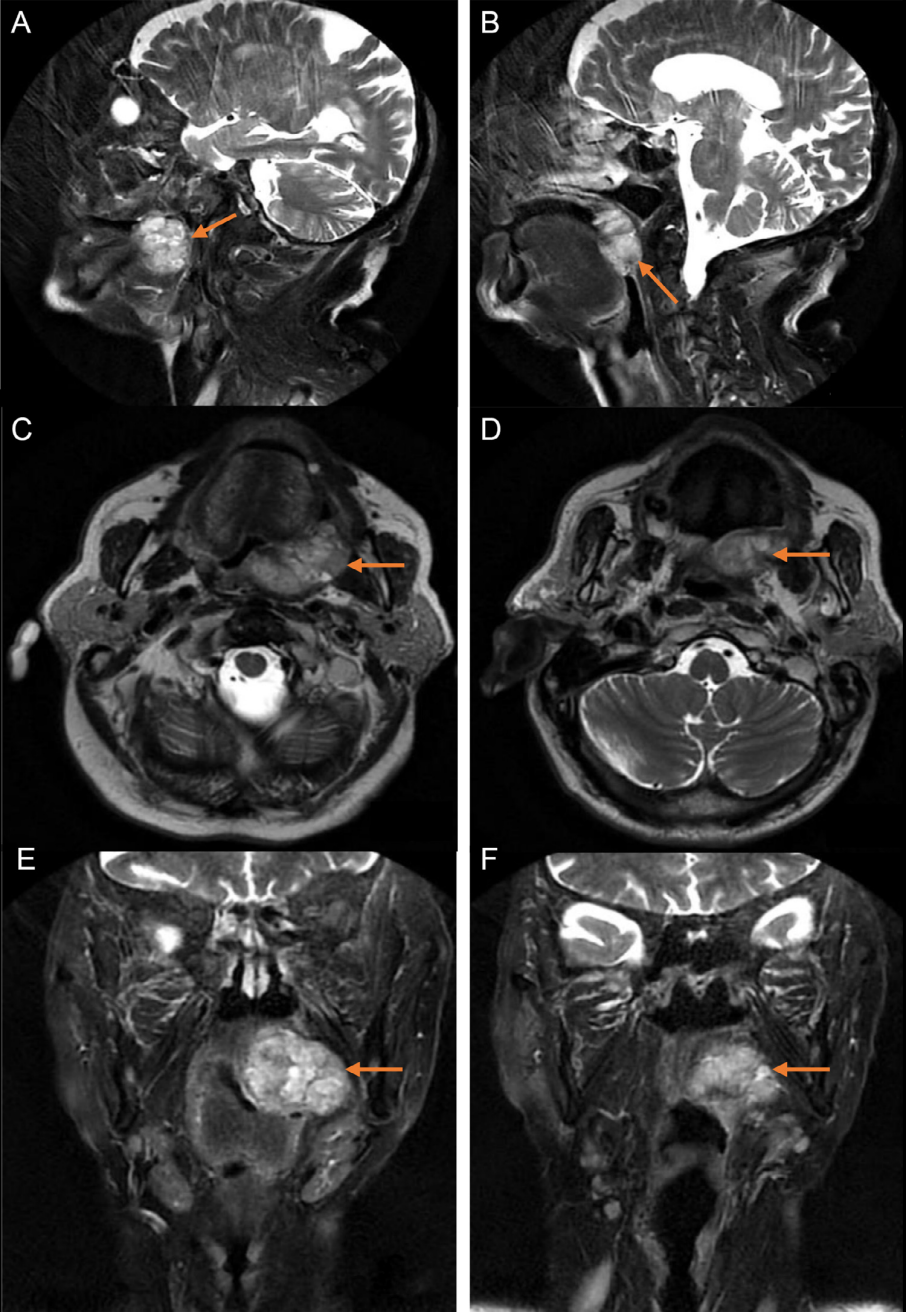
Initial head and neck MRI of our patient revealing a tumor of 39.4 × 21.4 × 39.7 mm (orange arrows). (A and B) Sagittal T2 propeller sequence. (C and D) Axial T2 propeller sequence. (E and F) Coronal T2 propeller sequence.
